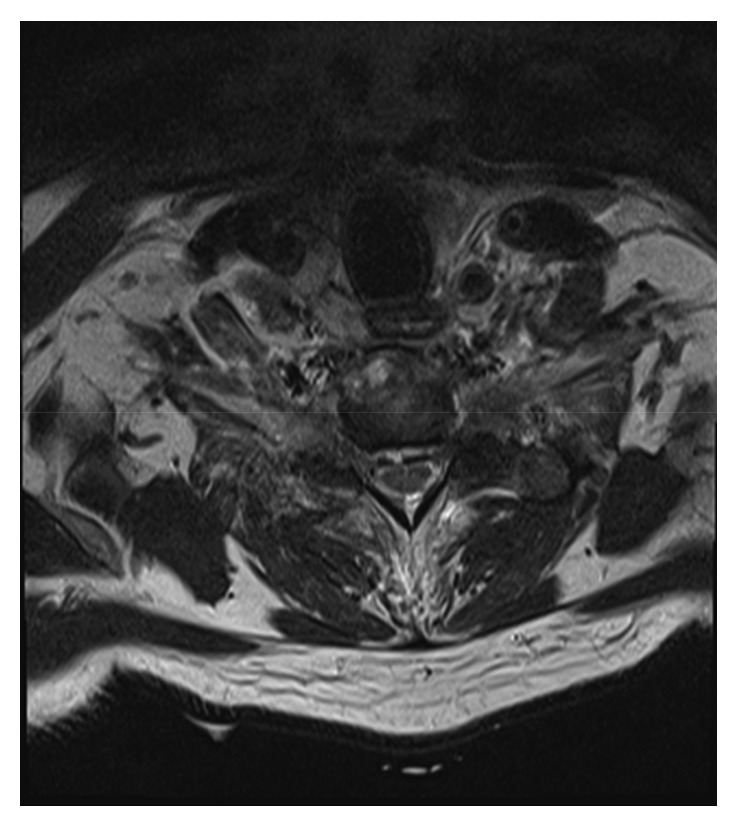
MRI thoracic spine showing local invasion of tumor.
